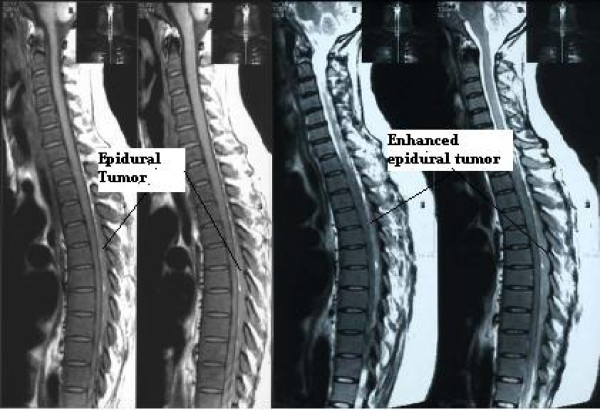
An MRI of the thoracic spine showed an extradural lesion extending from T3 to T11 (left) which became enhanced after a contrast injection (right).
